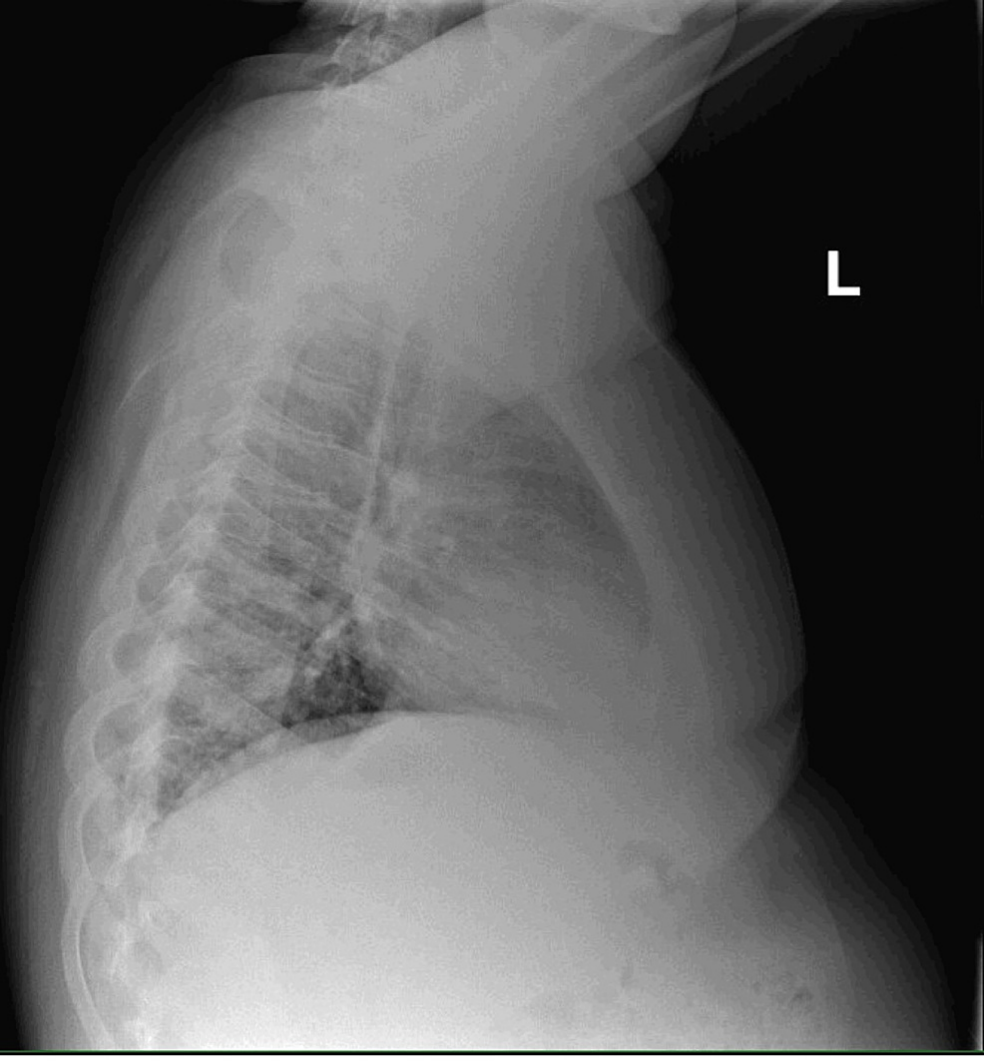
Lateral chest radiograph of the patient confirming an opacity in the right lower lobe.
